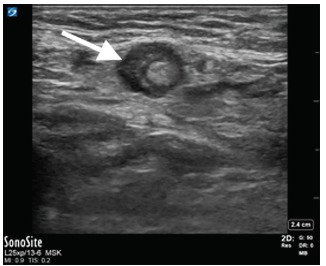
Point-of-care ultrasound image of appendix with hypoechoic rim (arrow).
